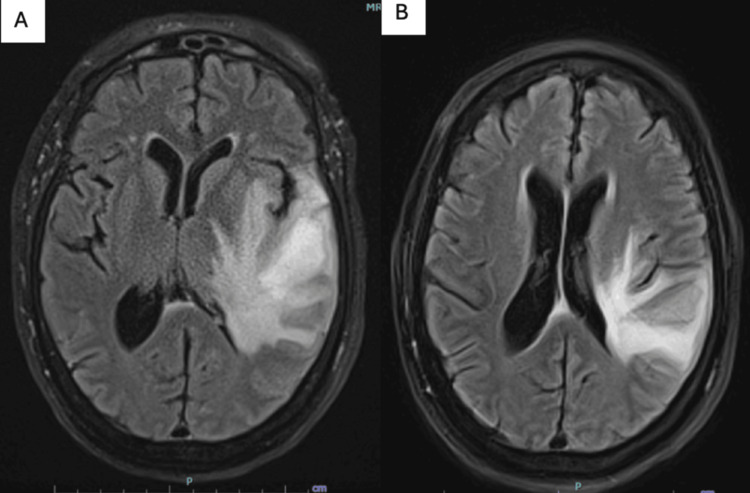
Repeated MRI imaging after two weeks (A) and three weeks (B) showing rapid improvement in the vasogenic edema on FLAIR FLAIR: fluid-attenuated inversion recovery
